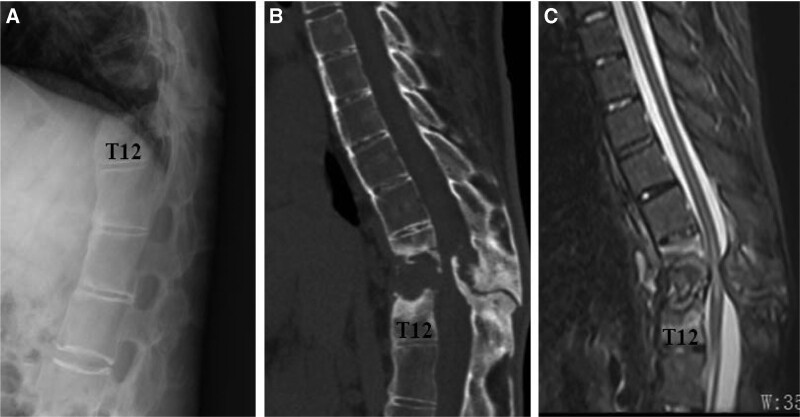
The X-ray (A), CT scan (B), and MRI (C) images depict syndesmophytes in the thoracic and lumbar regions, destruction of the T11 and T12 vertebrae, and extradural cord compression with myelopathic changes. CT = computed tomography, MRI = magnetic resonance imaging.
